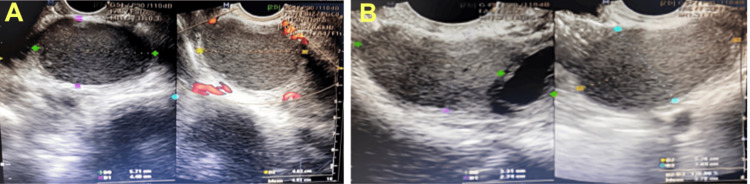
Transvaginal ultrasound findings: (A) A hypoechoic mass (44 × 57 × 46 mm) in the left ovary after three months of goserelin therapy; (B) A hypoechoic mass (45 × 33 × 27 mm) in the right ovary after three months of goserelin therapy
